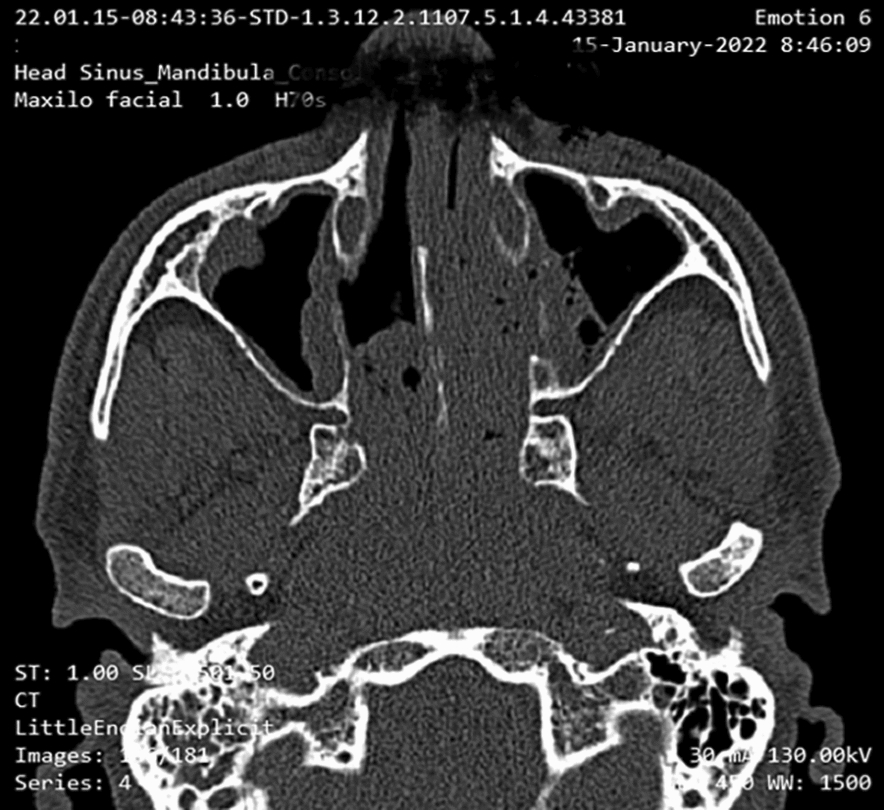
Computed tomography scan of the head: before treatment
